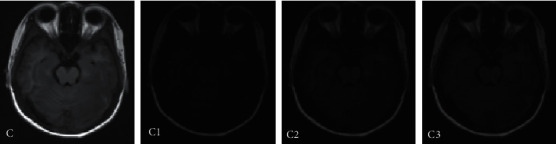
Comparison of MRI image segmentation at the bottom of the brain. Note: C represented the MRI image of the bottom of the brain, and C1, C2, and C3 referred to the results of MRI image segmentation by CNN segmentation, FCN segmentation, and multimodal CNN, respectively.
